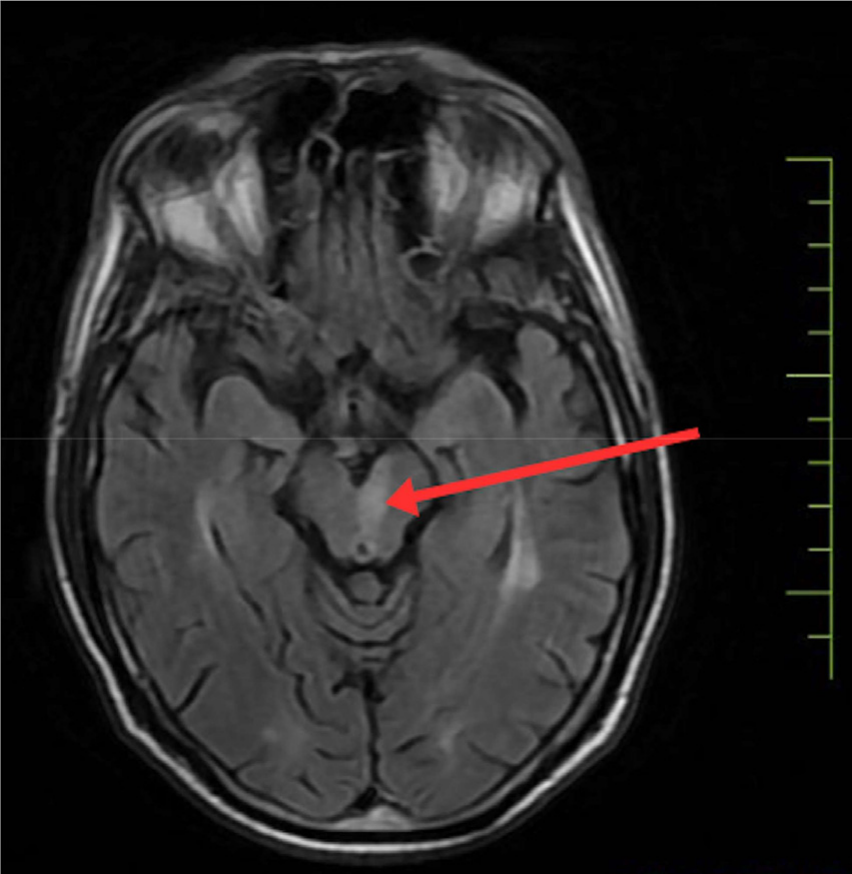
Shows the hyperintensity on the left paramedian midbrain in the axial section of the FLAIR view on MRI.
